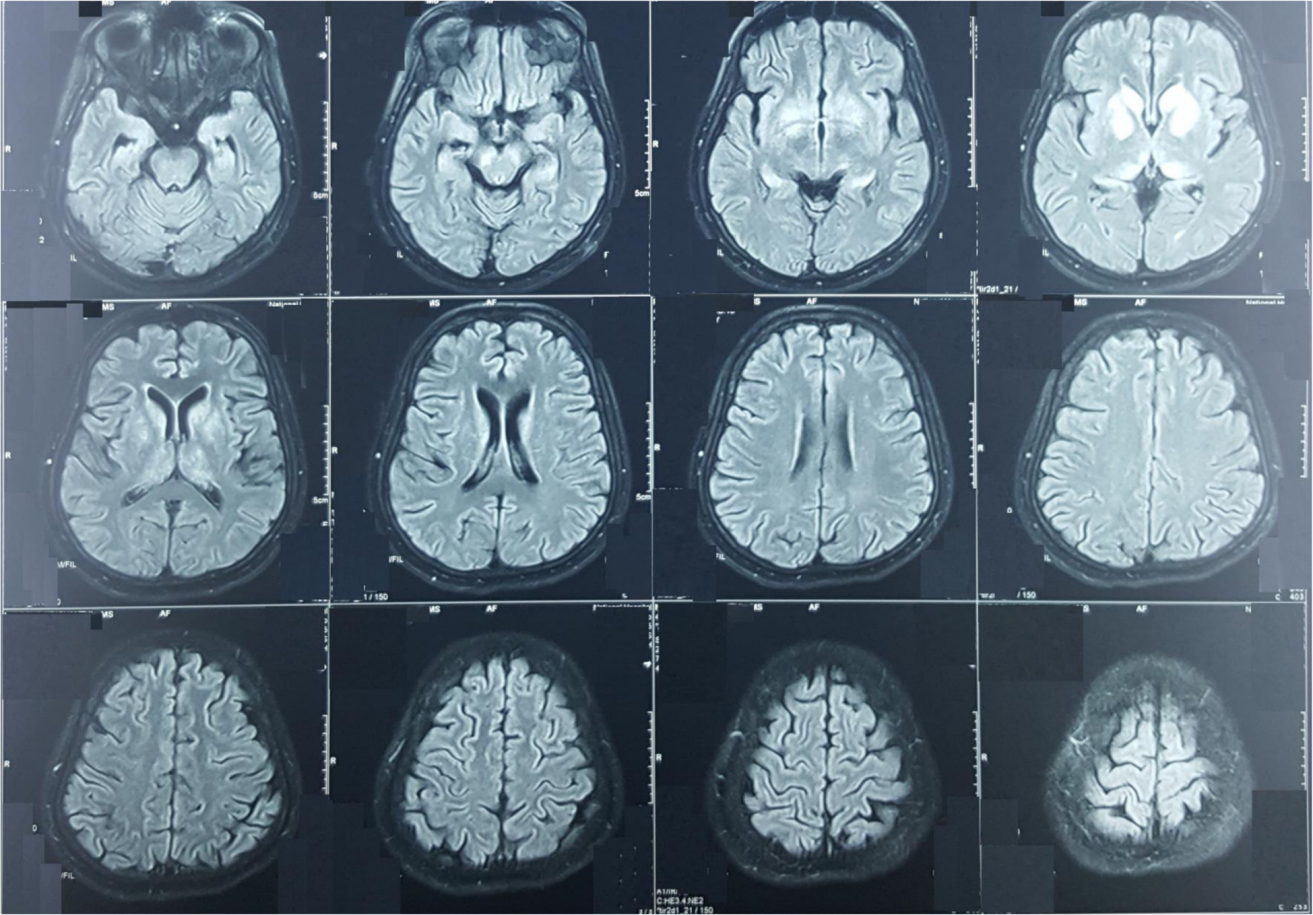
Magnetic resonance imaging of the patient’s brain showing symmetrical bilateral high signal intensities in basal ganglia, caudate head, and midbrain in fluid-attenuated inversion recovery
